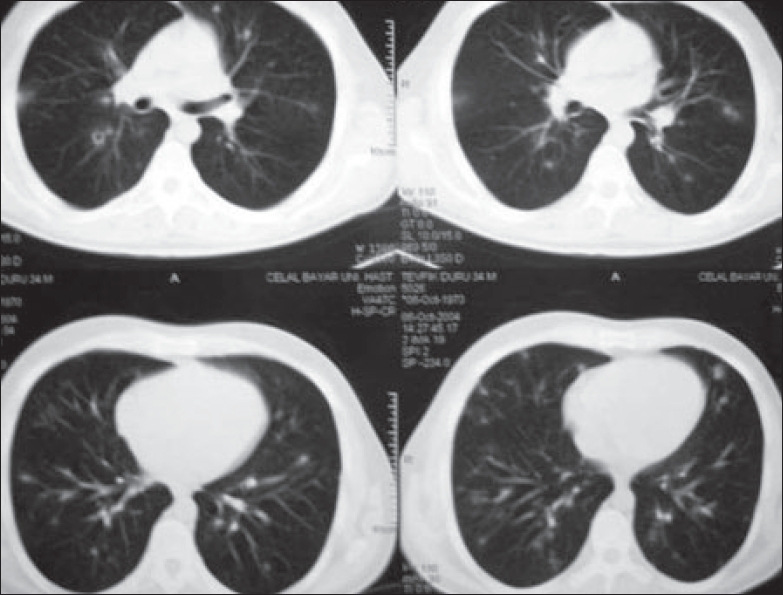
Thorax CT showing regression in the infiltrations
